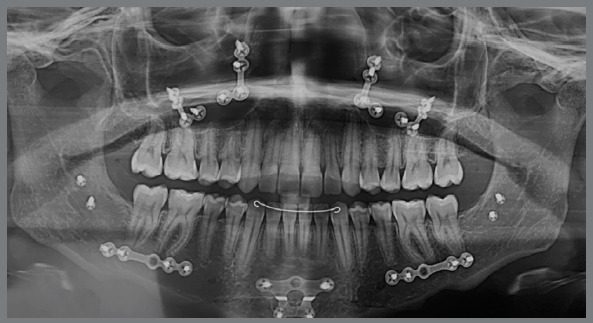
Final panoramic radiograph after treatment.
